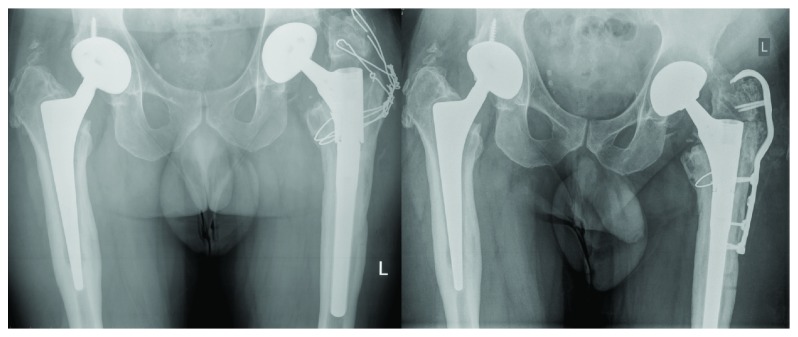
Radiograph a.p. view with broken stem and radiograph a.p. view of the second revision.
